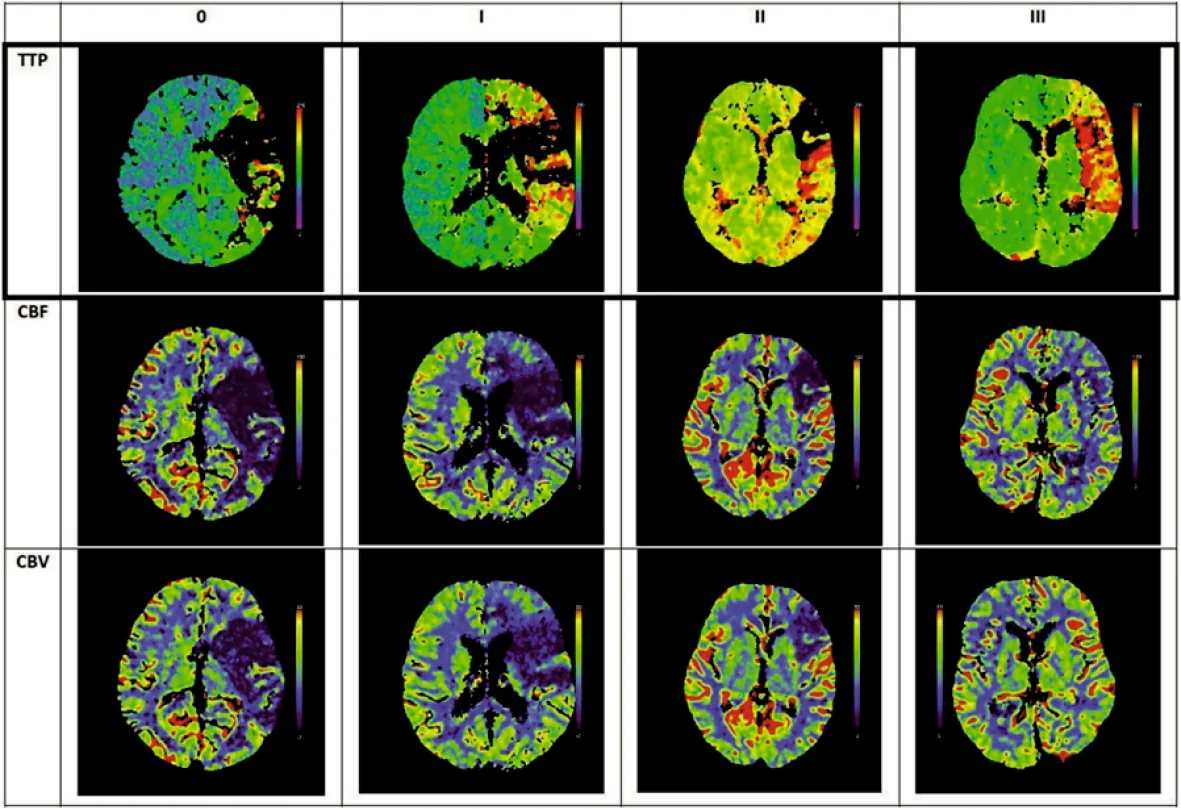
Examples of time to peak (TTP) based CT perfusion (CTP) maps divided into four categories, according to the percentage of »black hole« estimation in the hypoperfused region, with their corresponding cerebral blood flow (CBF) and cerebral blood volume (CBV) perfusion images.
